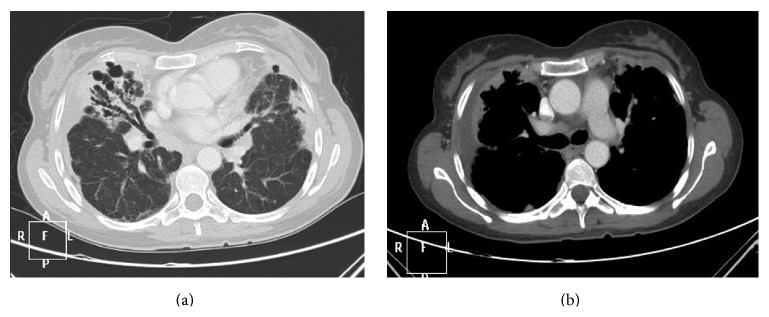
CT scan showing bronchiectasis in the medium and lower lobule and bilateral pleural thickening (a), without adenomegalies (b).
